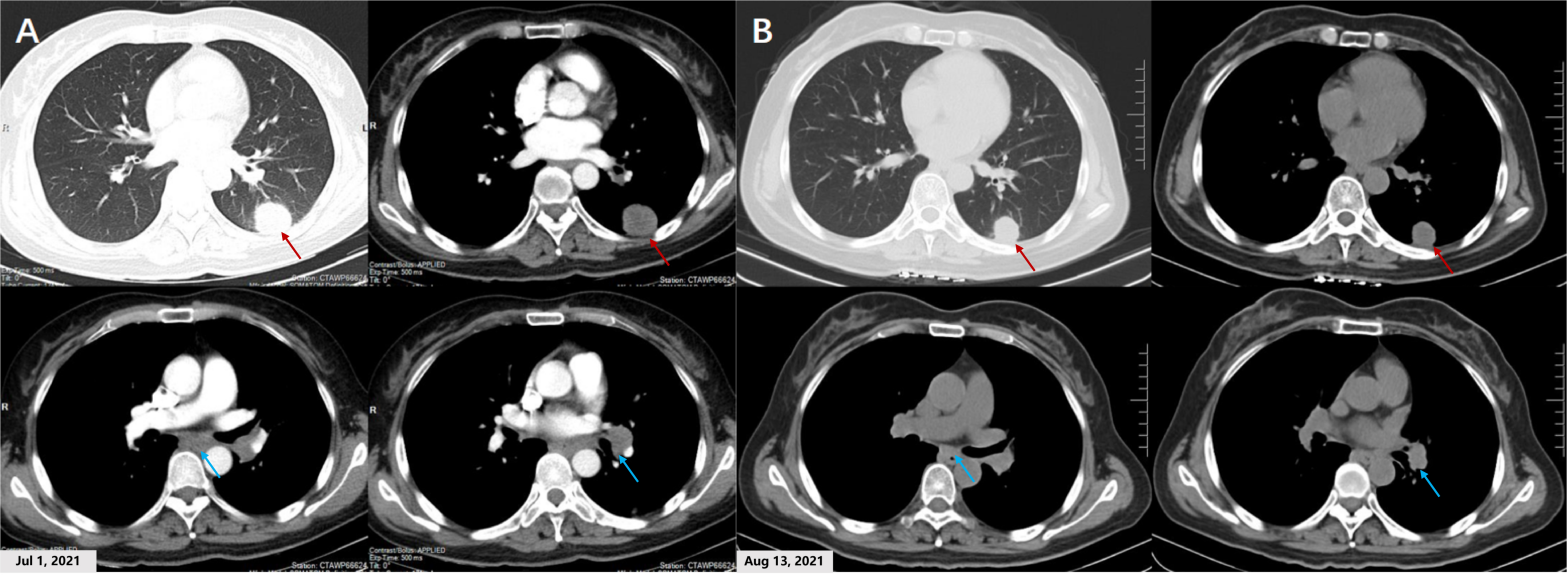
Chest CT scans of the patient before and after savolitinib treatment. (A) The chest contrast-enhanced CT scan on July 1, 2021 demonstrates a 3.8 cm × 2.9 cm abnormal lung mass (white arrows) in the lobe of left lung. Enhanced signal was not detected inside the mass. Left hilar and subcarinal lymphadenopathy (red arrows) also were observed. (B) After 21 days of savolitinib therapy, the chest CT scan on August 13, 2021 showed the mass (red arrows) had shrunk to 2.6 cm × 2.2 cm, achieving a partial response (PR). The Left hilar and subcarinal lymph node (blue arrows) also shrank significantly, and the short diameter did not exceed 7 mm.
